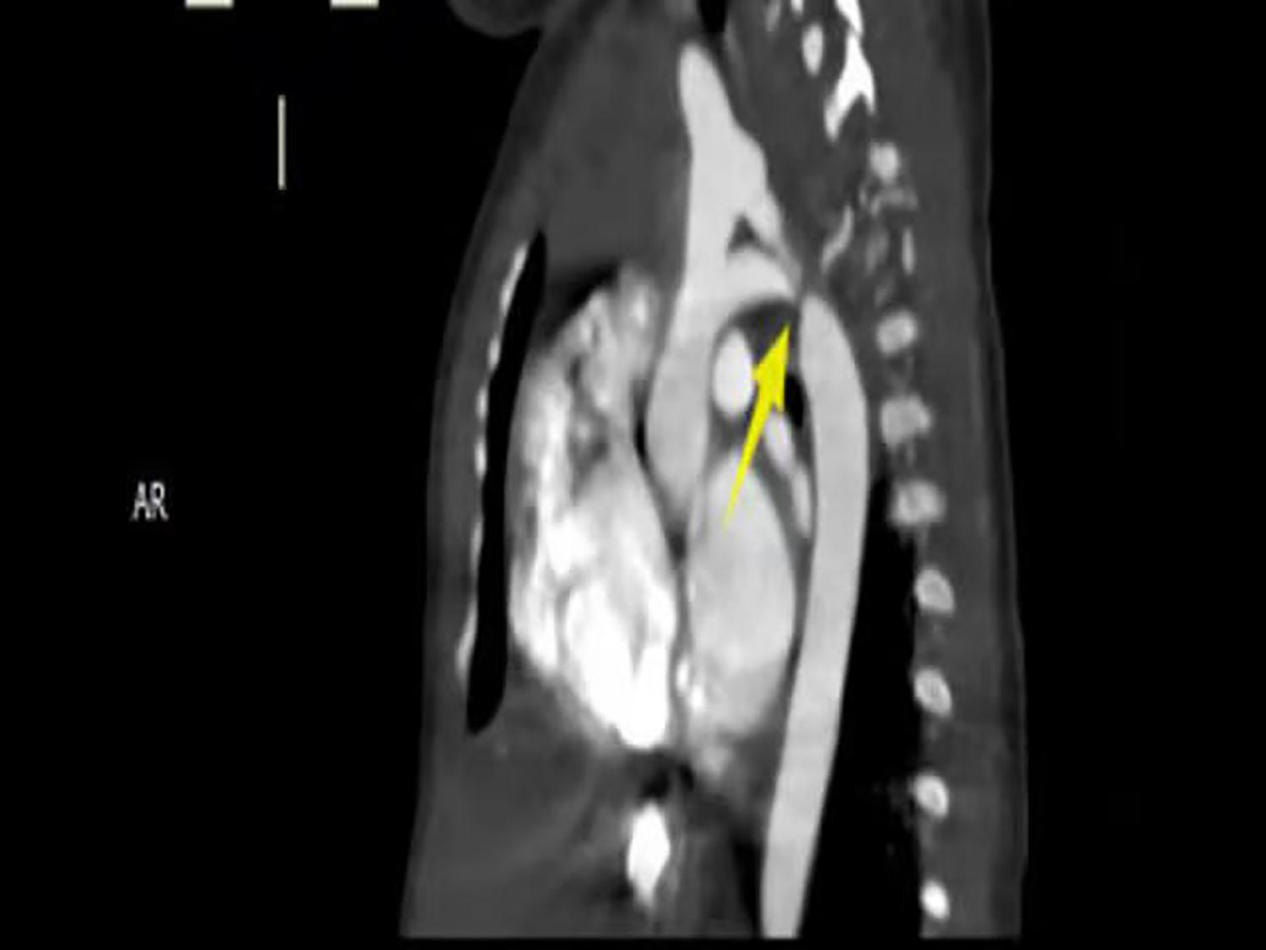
CTA: persistent fifth aortic arch associated with stenosis (indicated by arrows) and an interrupted fourth aortic arch. The fourth arch was interrupted at the distal end of the left subclavian artery, about 12 mm long. The fifth arch continued with the descending aorta, with a length of about 9 mm and an internal diameter of about 5 m at the proximal end.
